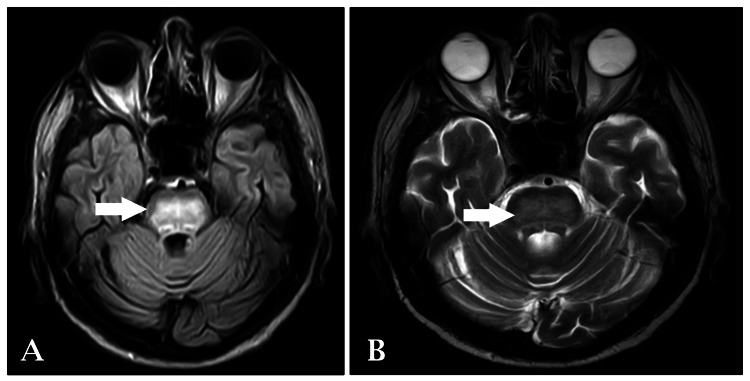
T2-weighted and FLAIR axial section of brain showing hyperintense lesion in pons extending to both sides of the midline FLAIR: fluid-attenuated inversion-recovery
